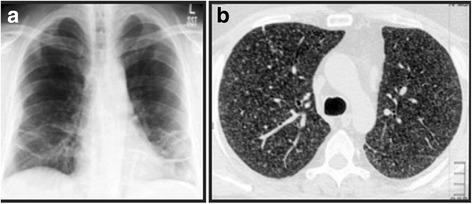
a Chest X-ray and b computed tomography scan of the chest showing multiple fine nodularities bilaterally in all lung fields
